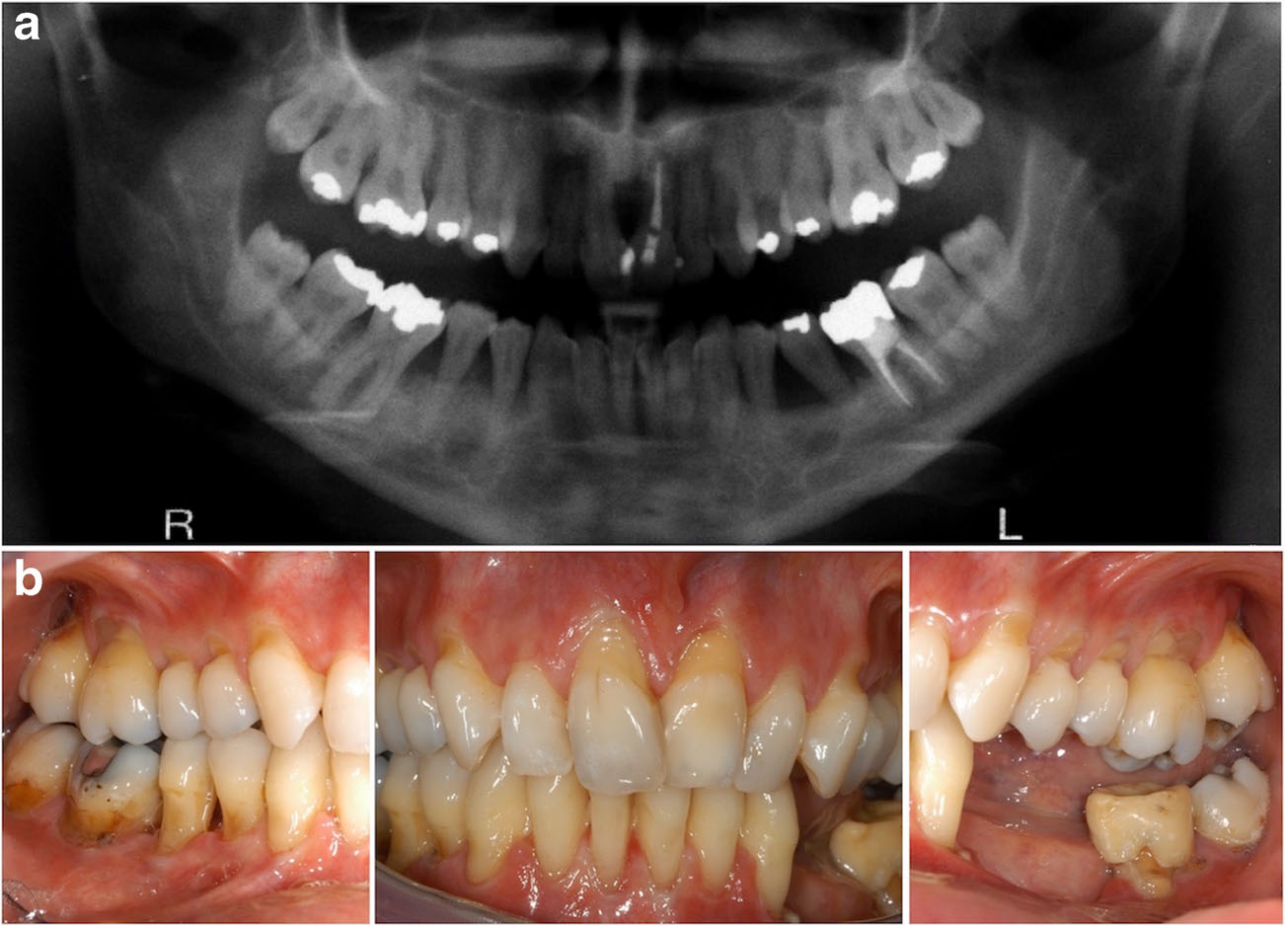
Oral features. a Intraoral radiograph of individual B:IV-10 at age 32 years. Notice severe periodontal bone loss in the lower jaw, especially teeth no. 44, 45, and 34, 35. b Intraoral photographs at age 34 years. Teeth no. 34 to 36 have been lost. Severe gingival recession (exposed tooth roots) and lack of attached gingiva with mucosa extending to the gingival margins is specific for periodontal EDS
